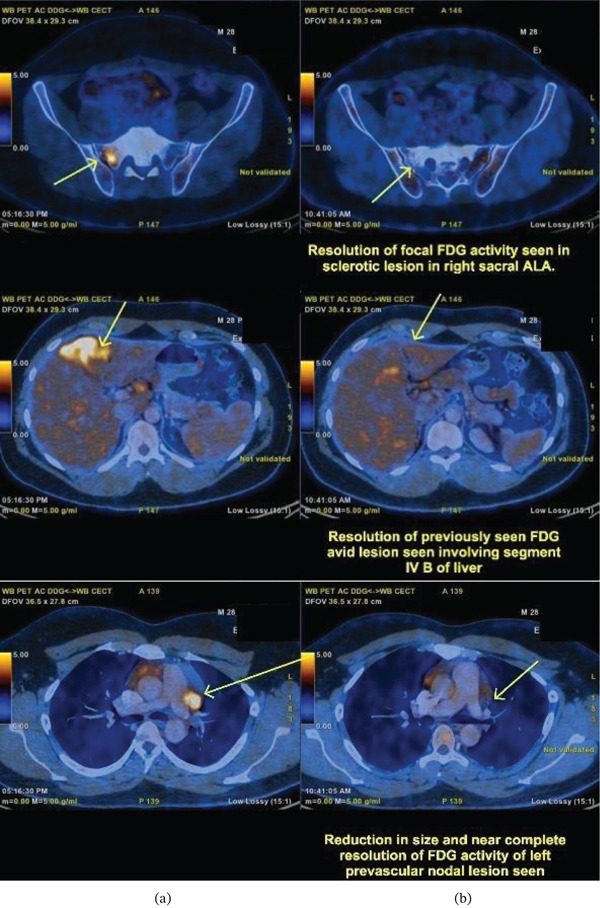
Response of PET‐CT scan of the patient showing comparison of (a) January 2024 scan images with (b) May 2024 scans. To note, brigatinib was started in February 2024 after the patient had progressive disease in the liver, nodes, and bones on alectinib.
